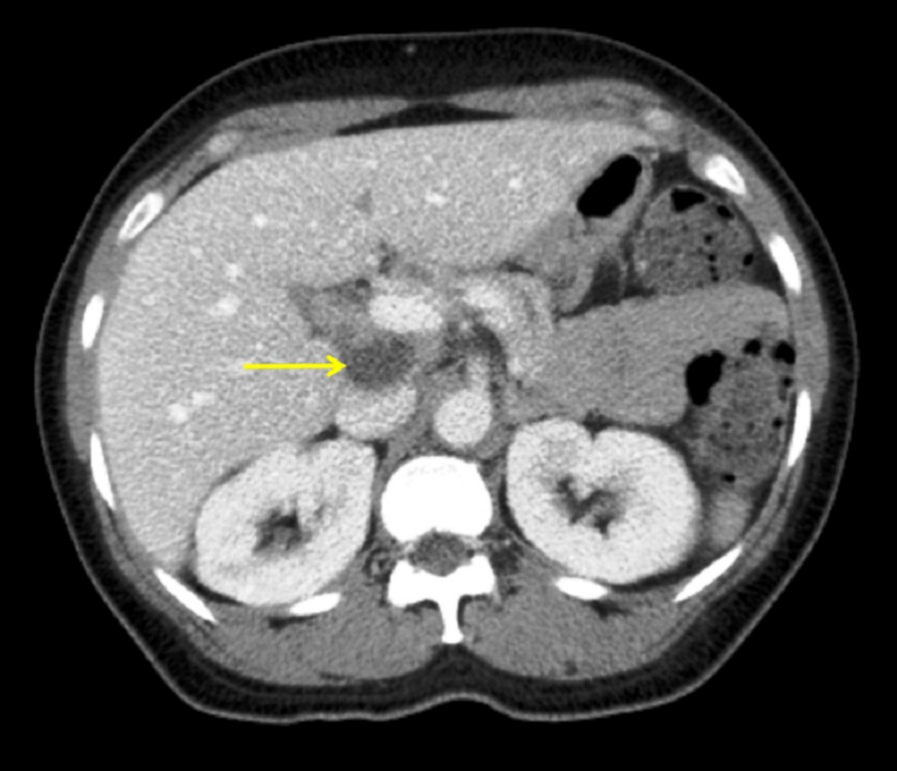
CT abdomen (transverse section) CT abdomen showing retrocardiac lymphadenopathy
